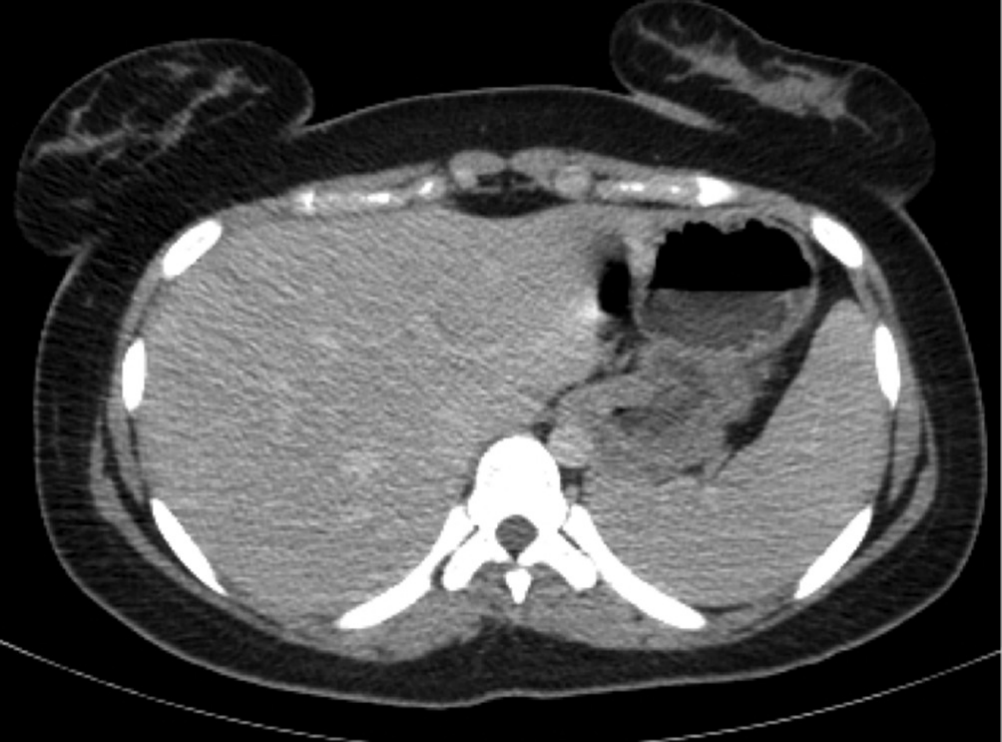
Corresponding contrast-enhanced CT image (axial section at the level of liver and spleen) showing normal splenic parenchyma without any focal nodular lesions.
